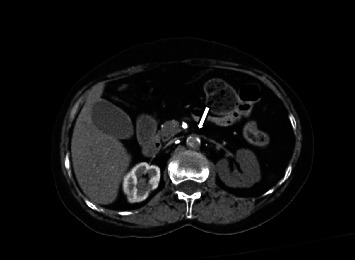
CT angiogram demonstrating AAO (arrow) with hypoperfusion of the left kidney.
